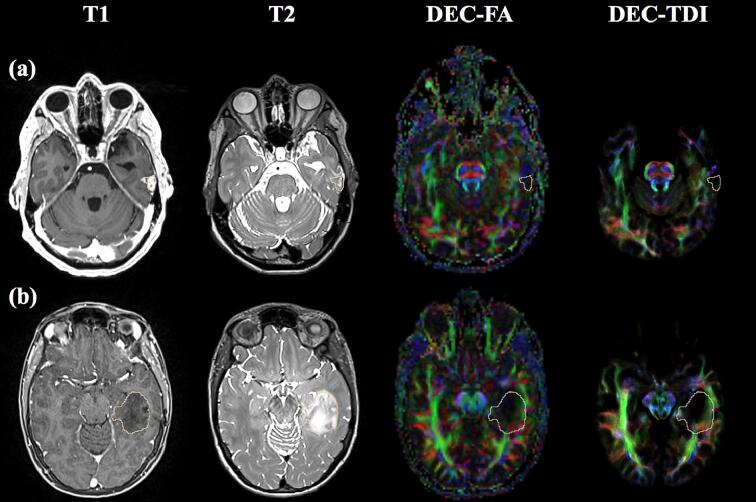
Example visualizations from two patients. (a) BTP 4, 39-year old female with glioblastoma, (b) BTP 12, 32-year old female with astrocytoma. Shown from left to right: single axial slices of the T1-weighted image, T2-weighted image, DEC-FA map, and DEC-TDI map. Tumor outlines are provided in pink on each image for reference. DEC = directionally encoded color, FA = fractional anisotropy, T1 = T1-weighted image, T2 = T2-weighted image, TDI = track density imaging. (For interpretation of the references to color in this figure legend, the reader is referred to the web version of this article.)
